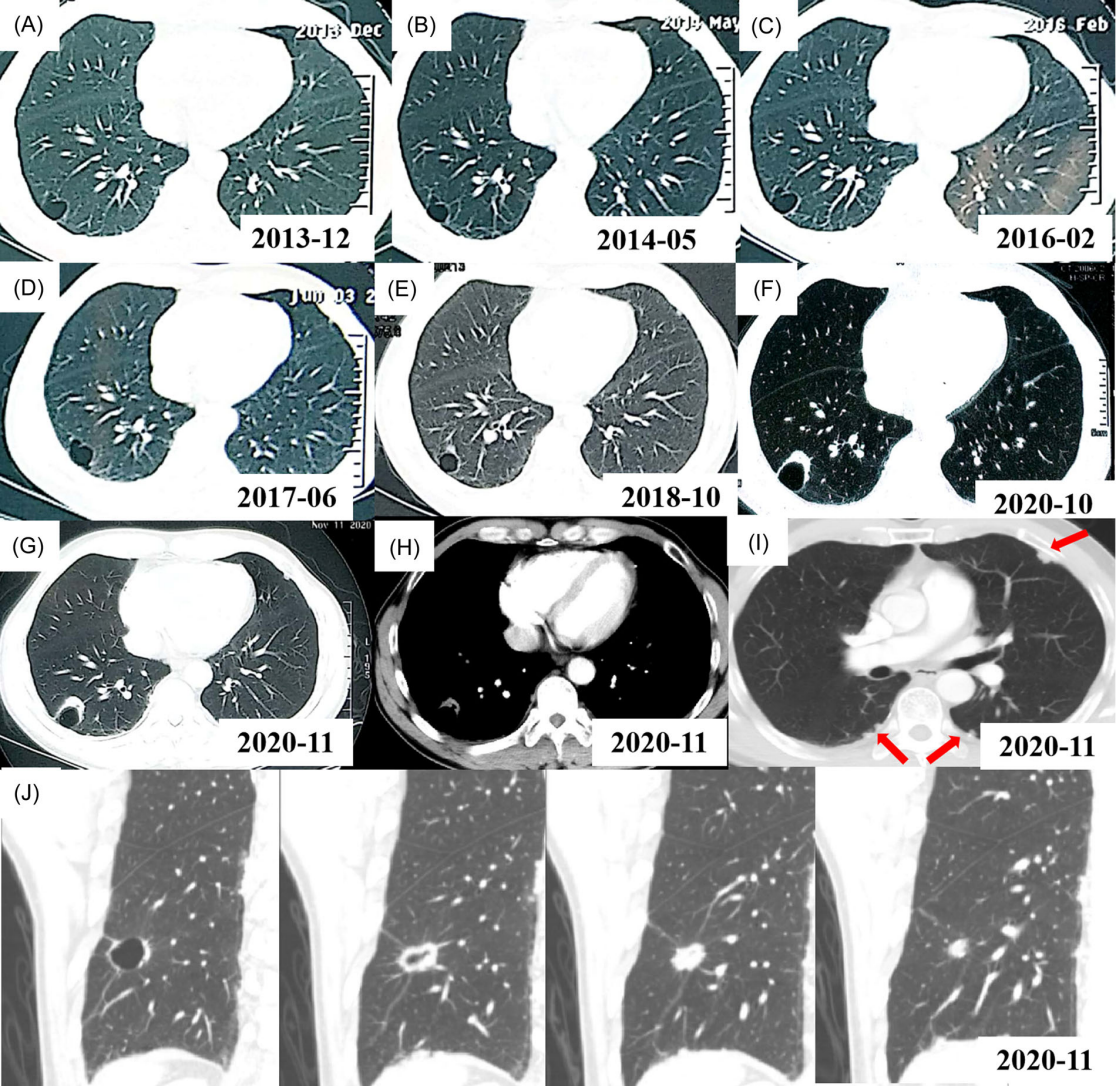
Computed tomography (CT) scans of the lung cystic airspaces from 2013 to 2020. (A) Initial CT shows a local thin‐walled air cavity in the right lower lobe in Dec 2013. (B–D) On a CT follow‐up, the size of the thin‐walled air cavity changed little in May 2014 (B), Feb 2016 (C), and Jun 2017 (D), respectively. (E) CT shows that a nonsolid nodule appeared adjacent to the wall of cystic airspace in Oct 2018. (F) CT shows that the size of the nodule increased and the wall of the cystic airspace thicken in Oct 2020. (G) CT shows a thicken‐walled cystic space with exophytic solid nodule along the cyst wall in Nov 2020. (H) CT shows a soft tissue nodule in the mediastinal window in Nov 2020. (I) CT shows local pleura irregular thickening and multiple small nodules on both lungs in Nov 2020. (J) CT images of the lung cystic airspace and nodule after three‐dimensional reconstruction in November 2020.
